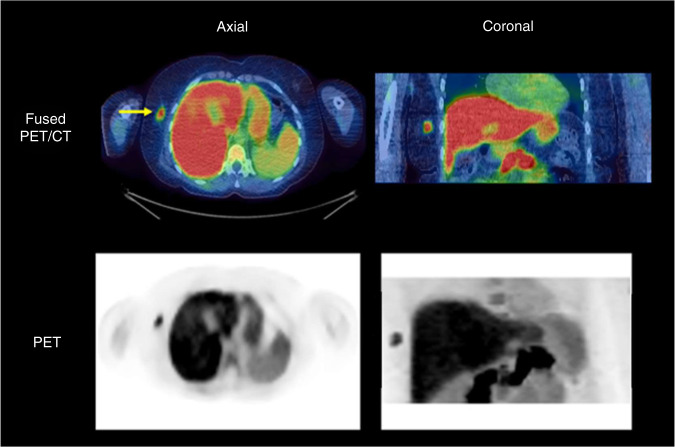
Summed 5- to 10-min dynamic ^18^F-fluciclovine PET and fused PET/CT images of a patient with oestrogen receptor-positive IDC. The primary tumour is indicated by the arrows in the axial plane of the fused PET/CT images.
